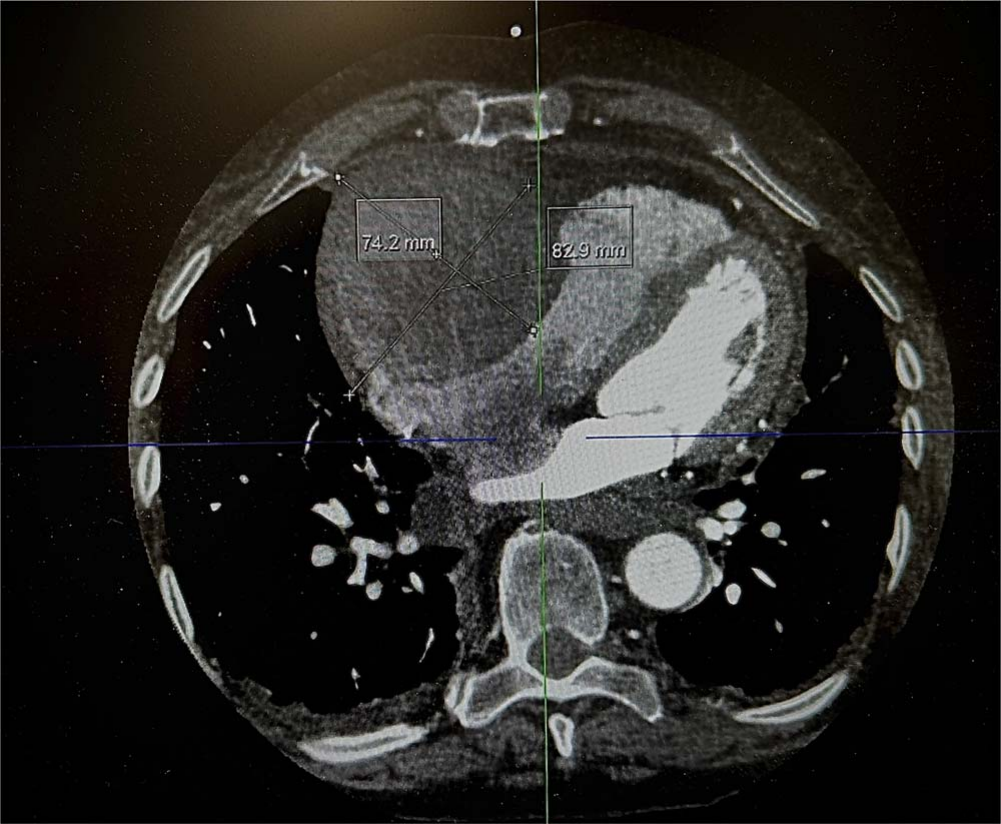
Axial plane of a contrast thorax CT scan showing the giant right coronary aneurysm.
